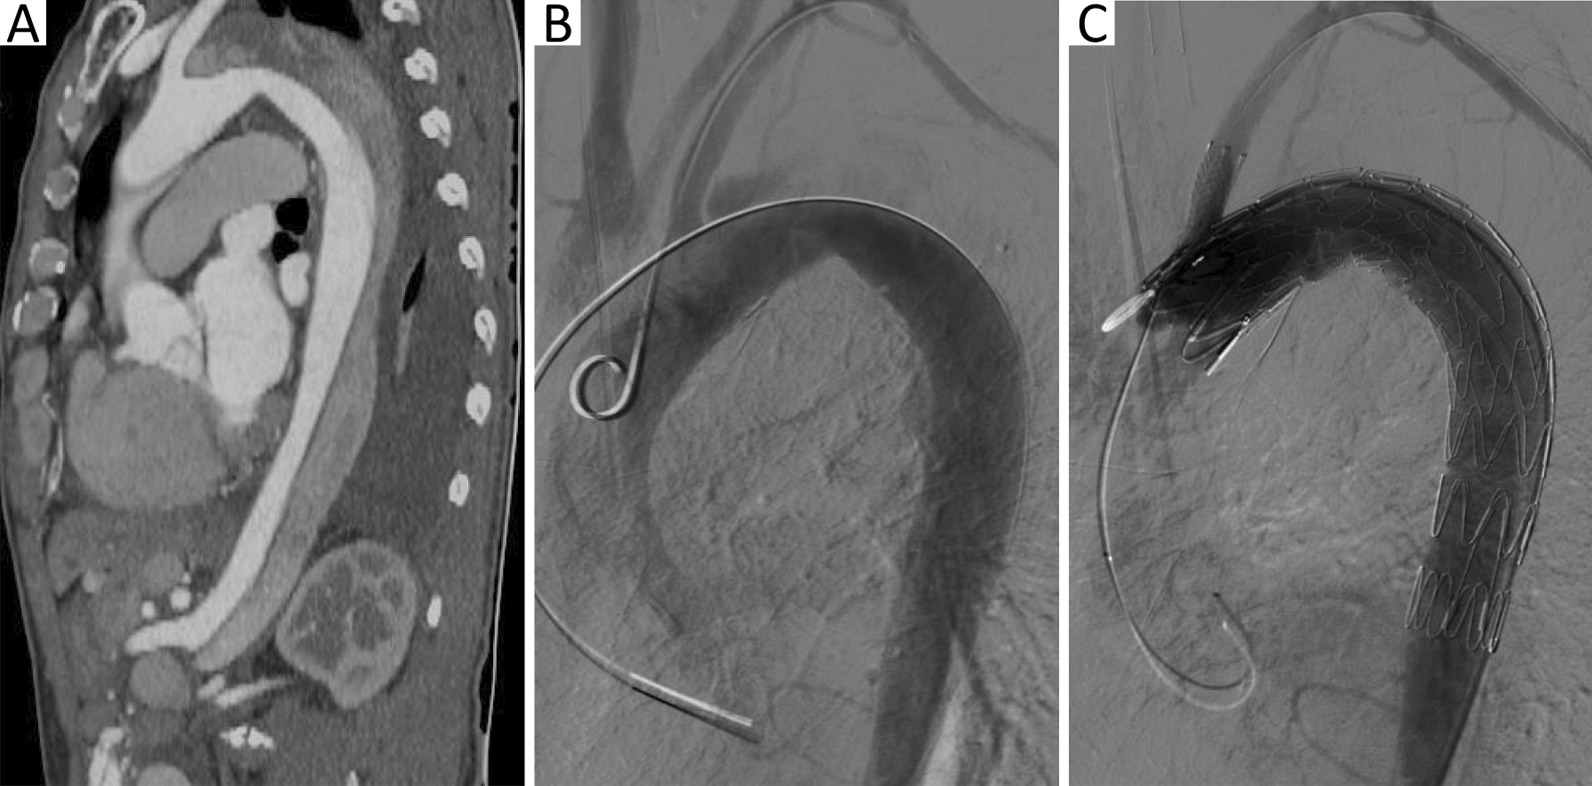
Historical images before admission. A CTA shows type B aortic dissection extending from the left subclavian artery to the bilateral iliac artery with partial thrombosis. B, C Images of angiography during TEVAR
